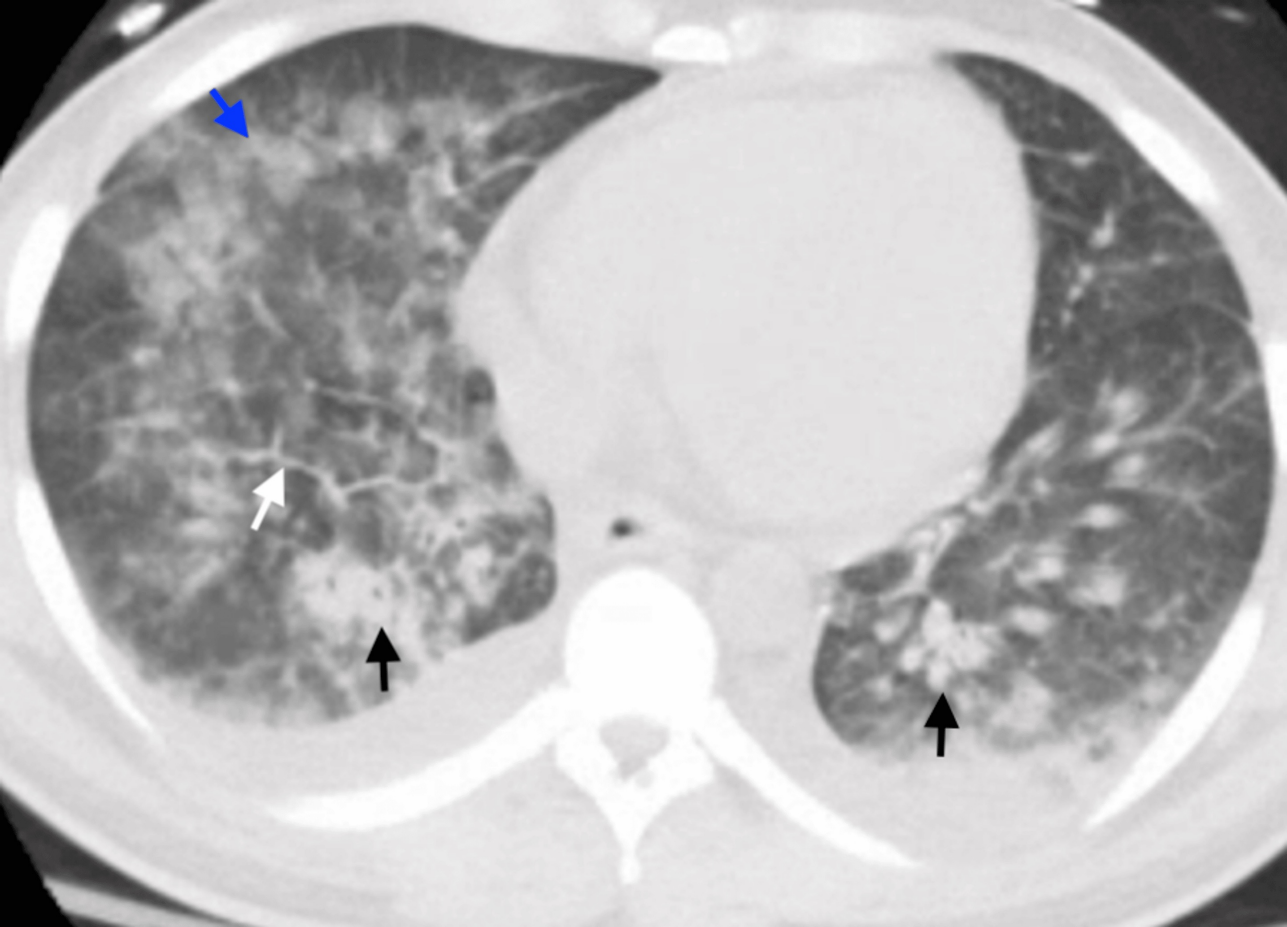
CT chest with contrast at time of respiratory decompensation This CT scan is notable for bilateral airspace consolidative opacities (black arrows), ground-glass opacities (blue arrow), and interlobular septal thickening (white arrow). These findings are most suggestive of pulmonary edema, but could also be seen with diffuse alveolar hemorrhage in the right clinical context. CT, computed tomography
